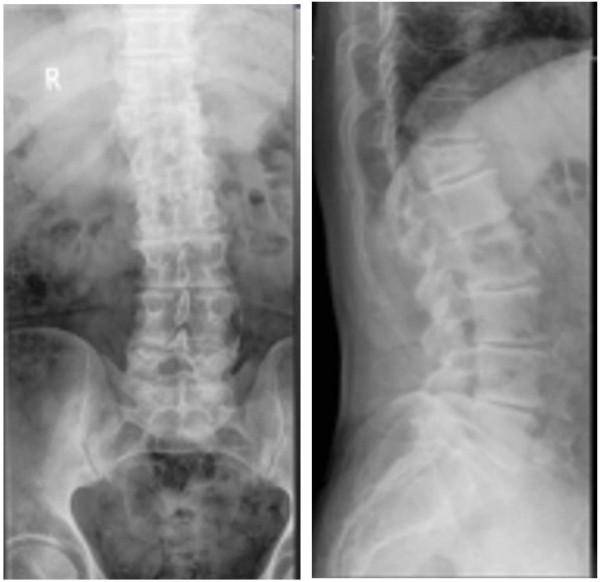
Plain radiographs obtained at baseline showing the patient’s T12 fracture in standing anteroposterior and lateral views of the lower thoracic and lumbar spine.
